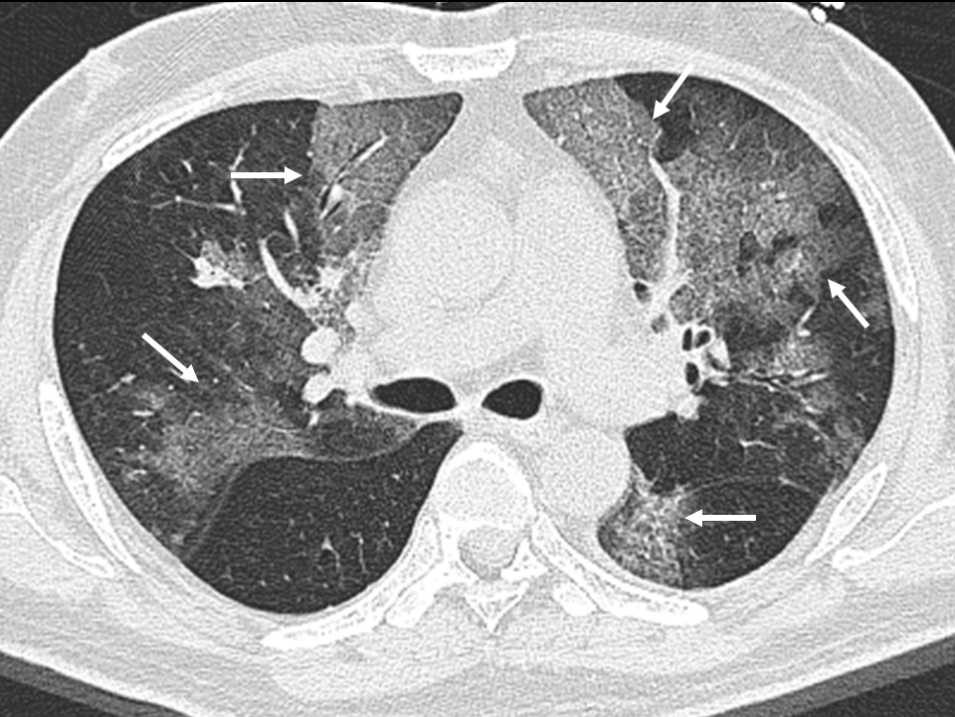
Non-contrast high resolution computed tomography axial image of the thorax with white arrows showing bilateral, circumscribed areas of ground-glass opacification in both lungs with associated reticular changes.
